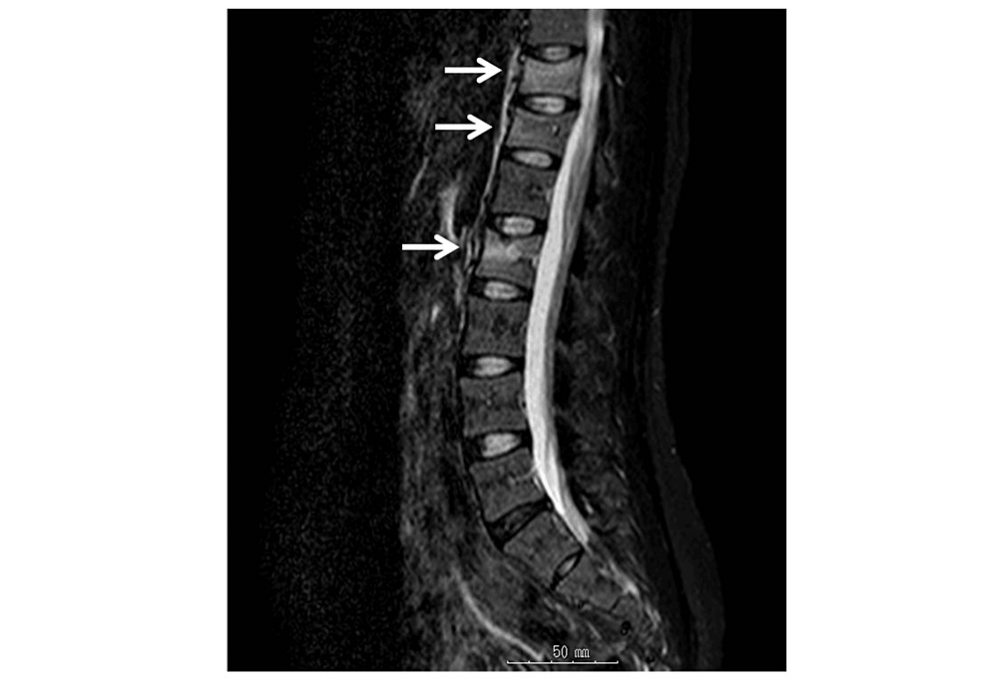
Magnetic resonance imaging (MRI) findings at pregnancy and lactation-associated osteoporosis (PLO) diagnosis. Multiple compression fractures of the 11th and 12th thoracic vertebrae, and second lumbar vertebrae were noted.
